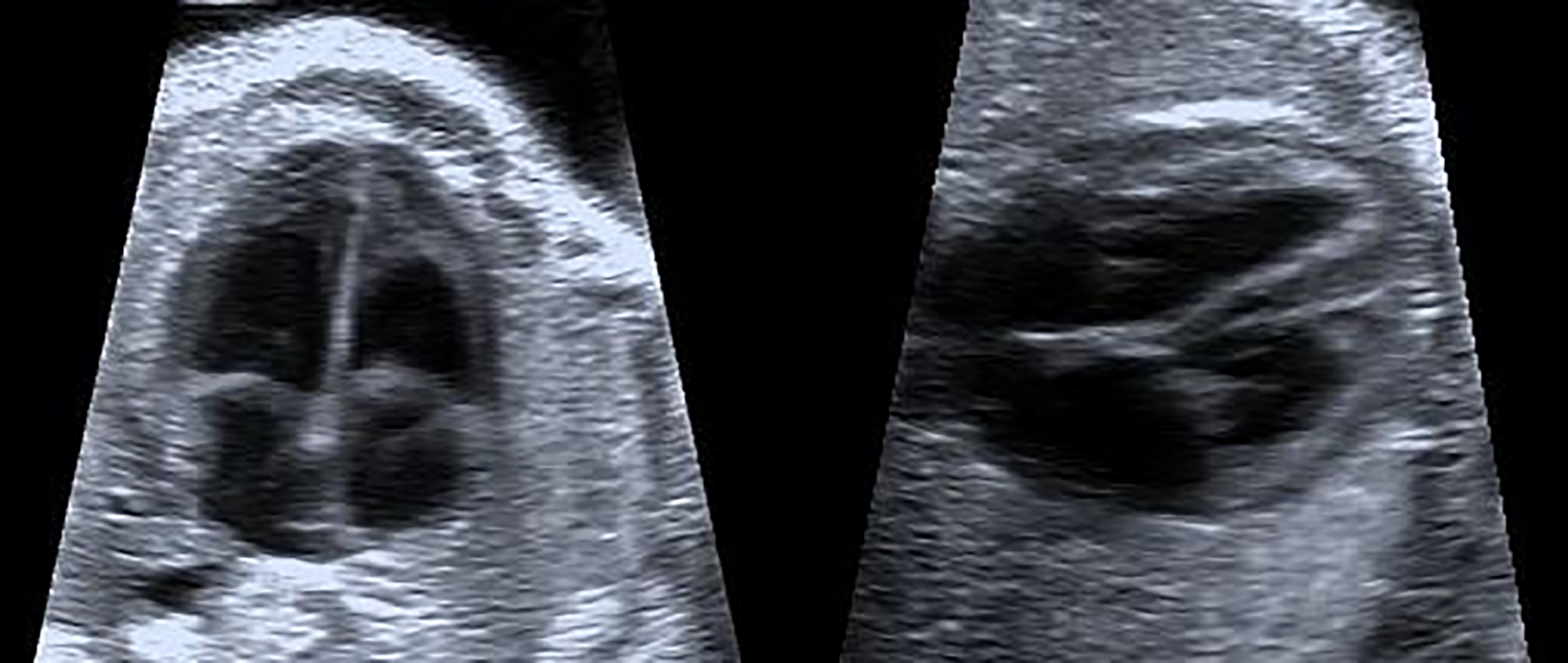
Example of a four chamber view of a fetal heart in longitudinal or apical (left) and transverse (right) section. Note that the interventricular septum and especially the thin interatrial septum are displayed best in the “in plane-view” of the transverse view where the ultrasound waves meet those structures perpendicularly.
